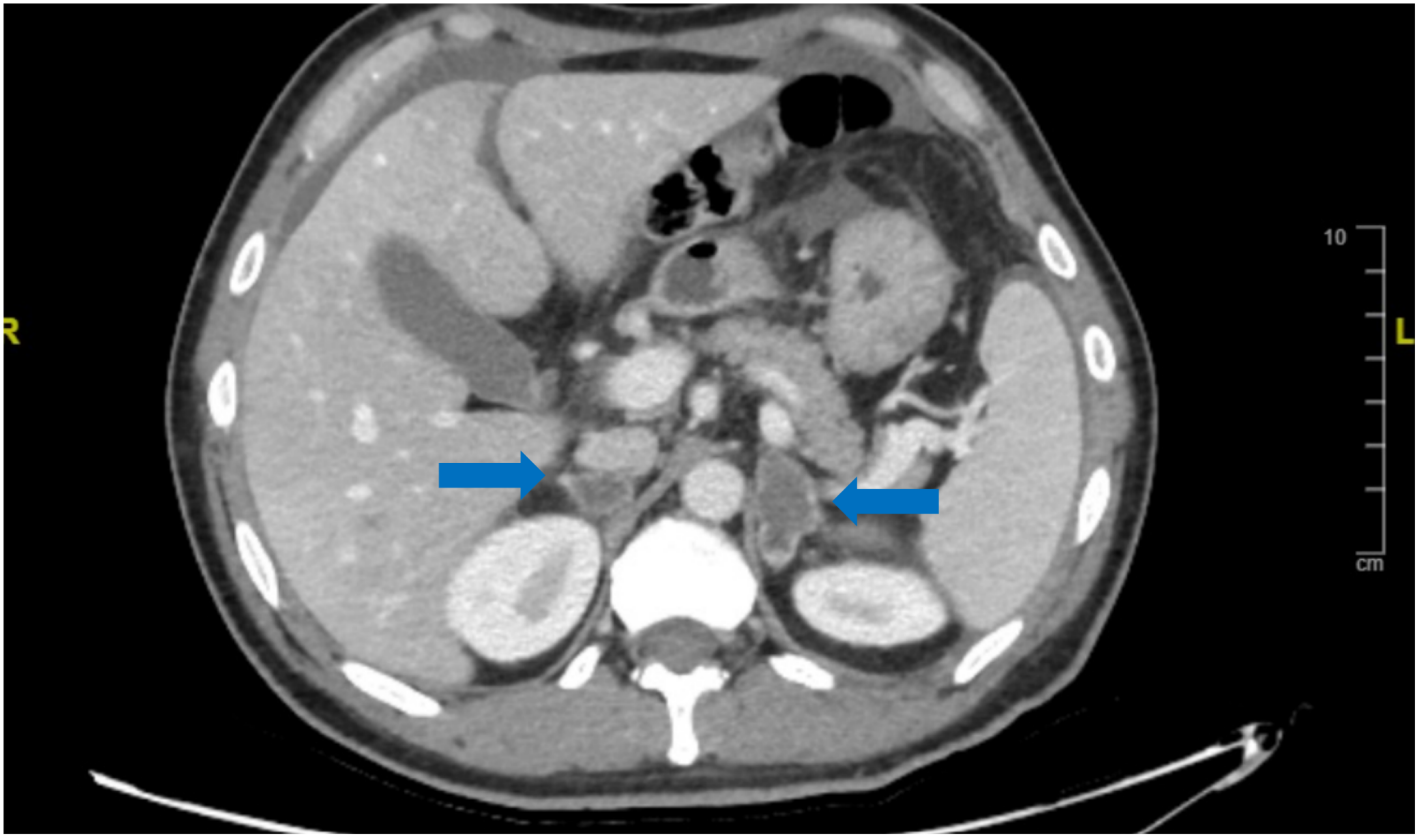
CT scan showing an acute increase of the dimensions (blue arrows) of both adrenal glands in case 1.
